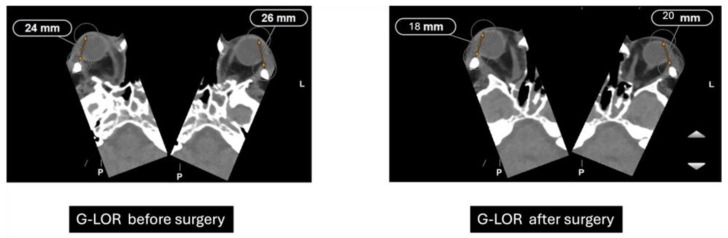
Preoperative and postoperative CT measurements of G-LOR (Globe-Lateral Orbital Rim) distance. Axial CT scans showing a reduction in proptosis following orbital decompression surgery. Preoperative G-LOR values measured 24 mm (right) and 26 mm (left), while postoperative measurements decreased to 18 mm (right) and 20 mm (left), indicating effective posterior repositioning of the globe.
